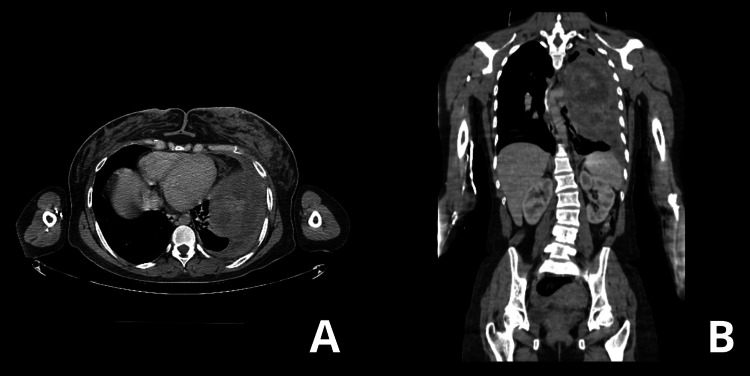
Follow-up computed tomography A, B: A known heterogeneous lesion is shown in the left lung, displacing the mediastinum to the right. The lesion has dimensions of 14.7 cm x 11.5 cm x 18.4 cm with mass effect.
